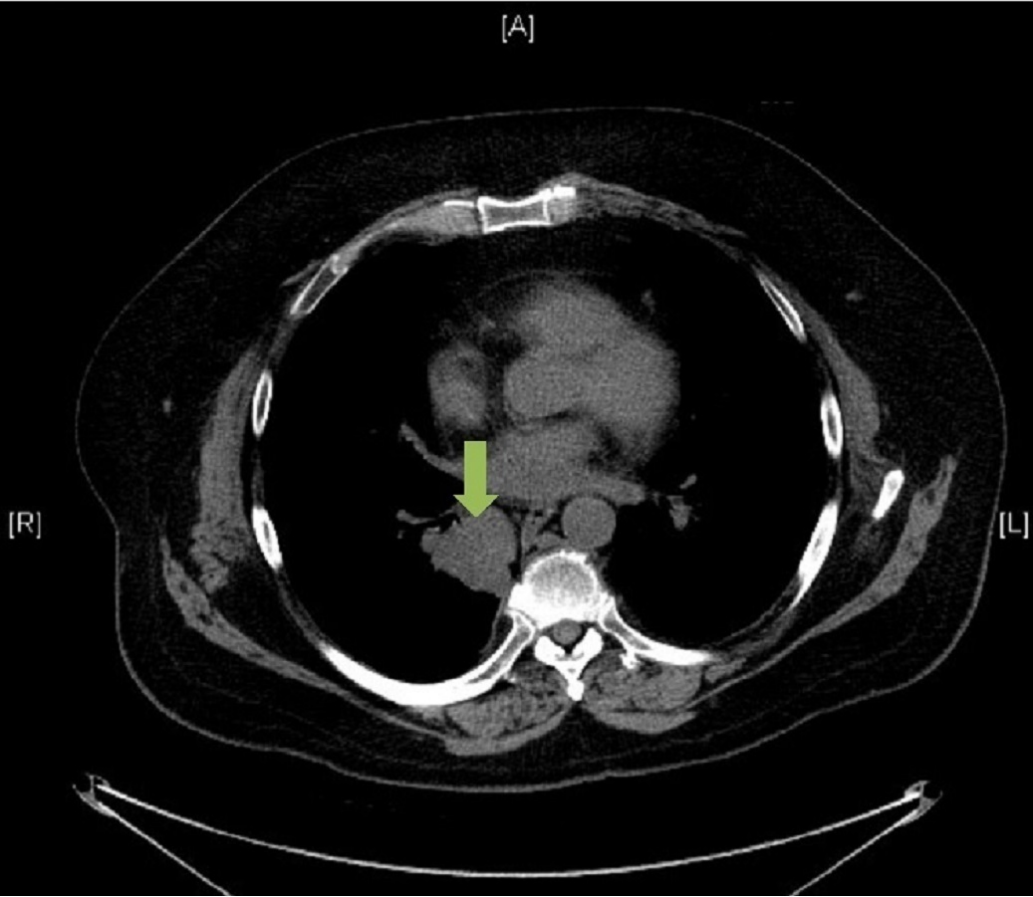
Preoperative CT of the Thorax Axial slice. Contrast-enhanced image that demonstrates a 2.1 cm primary papillary adenocarcinoma of the right lower lobe of the right lung.
